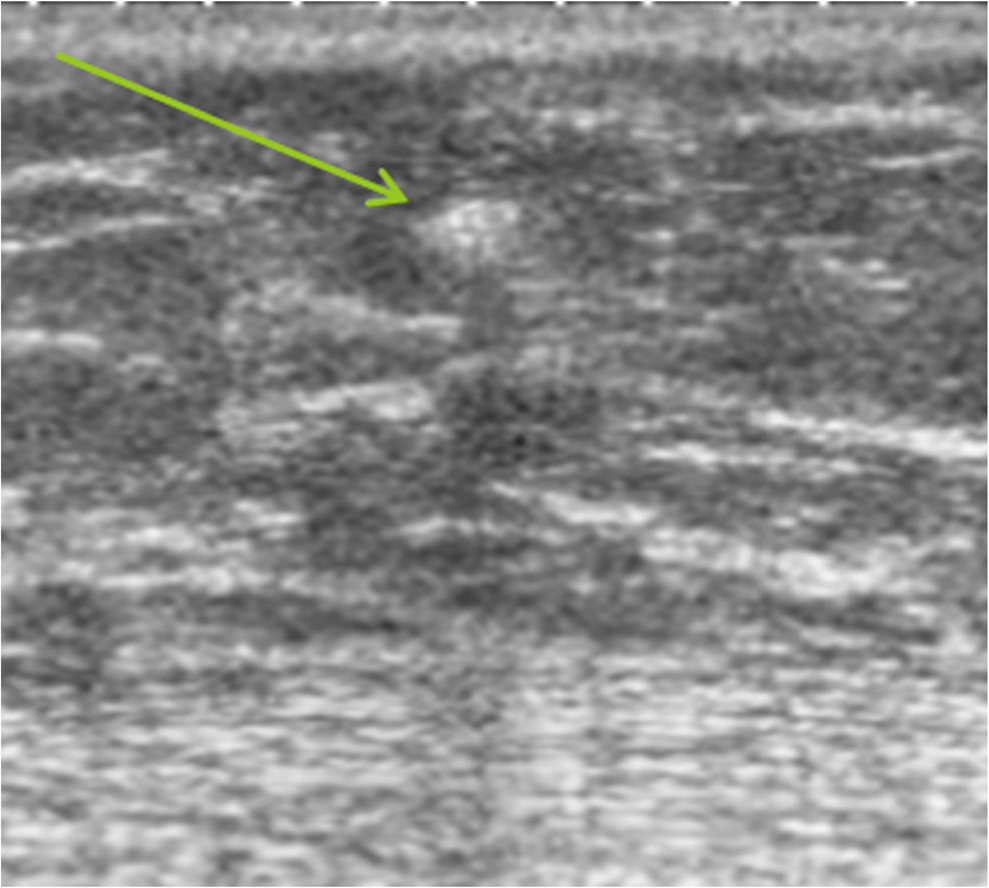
Metastasis. Ultrasound showing a small hyperechoic, attenuating mass (*arrow*) in a patient followed for malignant melanoma. Cytological examination after ultrasound-guided fine-needle aspiration: very numerous spindle-shaped, pigmented, round malignant cells, corresponding to a metastasis from known malignant melanoma in this patient
